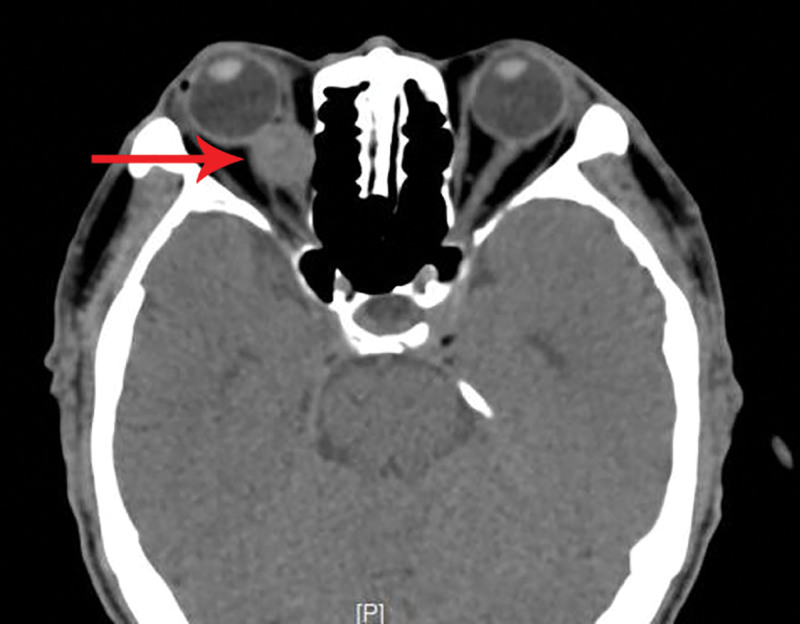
CT reveals a soft tissue mass in the retrobulbar space of the right eye. CT = computed tomography.
